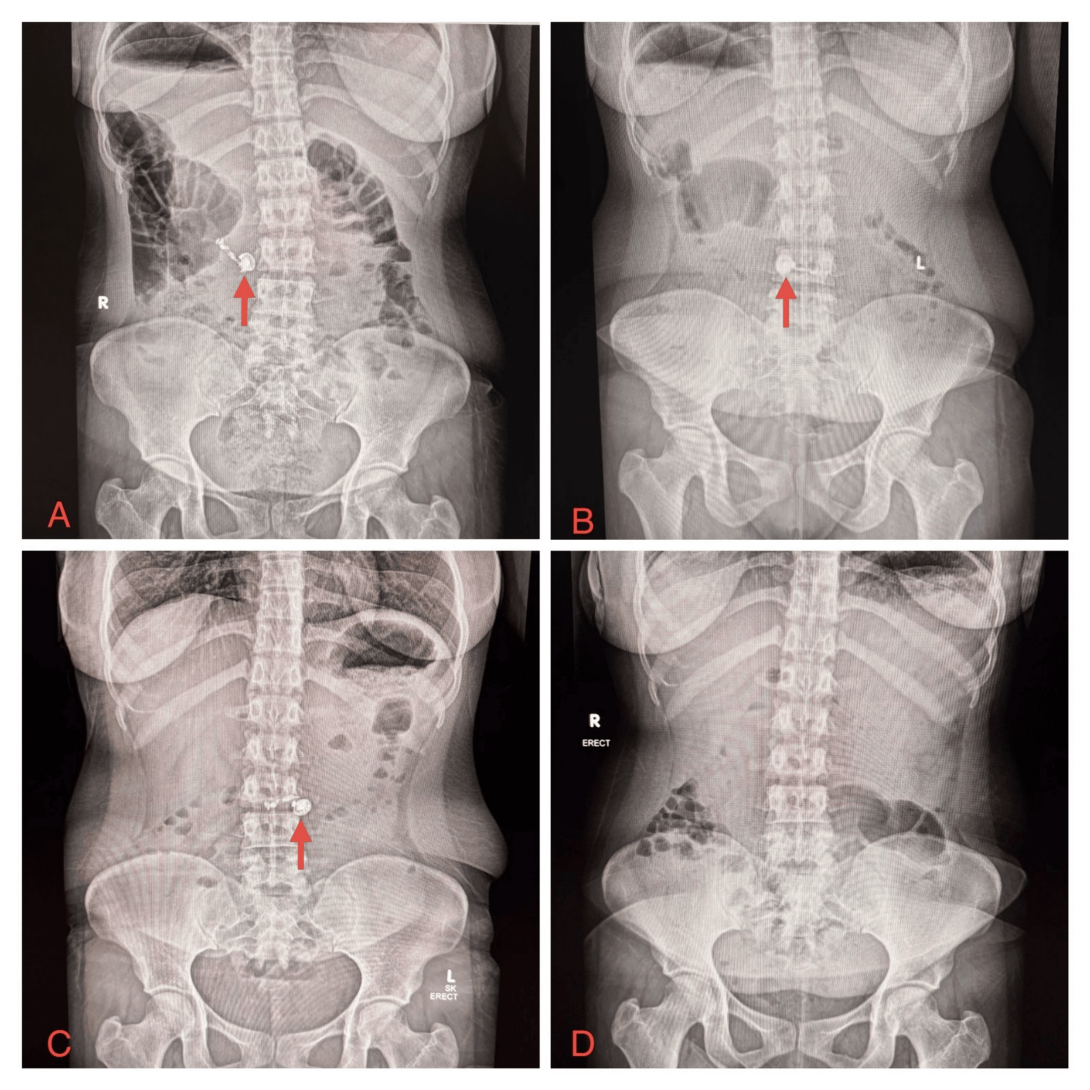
Serial erect abdominal radiographs demonstrating the progression and spontaneous passage of an ingested wireless earbud (A) Erect abdominal radiograph obtained at presentation demonstrating a single radiopaque foreign body consistent with a wireless earbud projected over the second part of the duodenum (red arrow). (B) Repeat erect abdominal radiograph at six hours post-ingestion demonstrating distal migration of foreign body to the region of the duodenojejunal junction (red arrow). (C) Follow-up erect abdominal radiograph at twelve hours post-ingestion demonstrating transient stagnation of the foreign body within the proximal jejunum (red arrow). (D) Follow-up erect abdominal radiograph on day five post-ingestion demonstrating complete passage of the foreign body, with no residual radiopaque density. R, right; L, left; SK, skull-knee direction of the X-ray beam.
